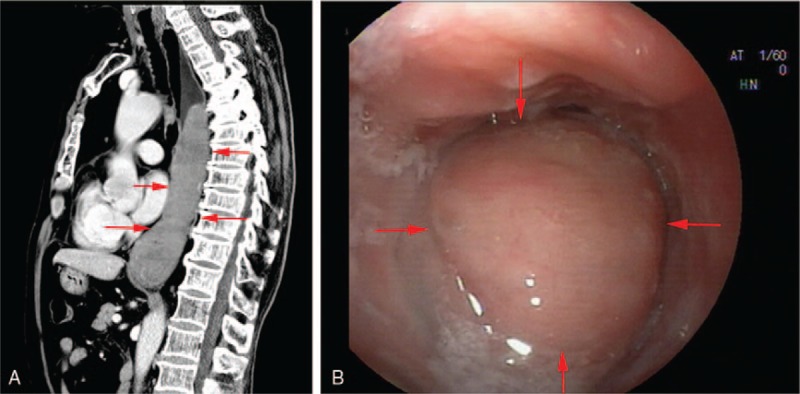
Representative images of computed tomography (CT) scan and endoscopic examination. (A) CT scan showed a huge well-circumscribed and homogeneous ellipsoidal mesenchymal neoplasm in the middle and lower esophageal section. (B) Endoscopic examination revealed an oval shaped lesion protruding in to the esophageal lumen with smooth-surface starting at 20 cm from the incisor teeth and extending up to cardia.
